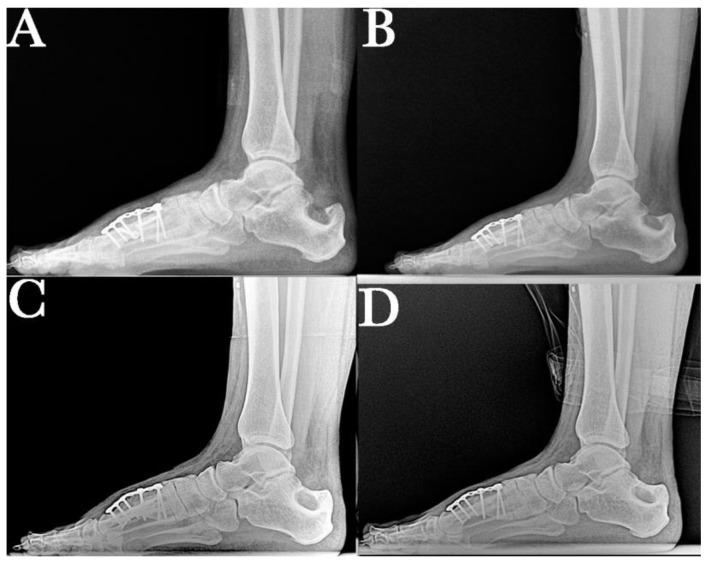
Correct position of the first metatarsal in the transverse plane and the evolution of the osseointegration process of an autologous graft. Images at (A) 6-month, (B) 12-month, (C) 18-month, and (D) final 24-month follow-up after surgery, showing bone callus consolidation and remodeling, and maintaining correction of the analyzed radiographic parameters.
